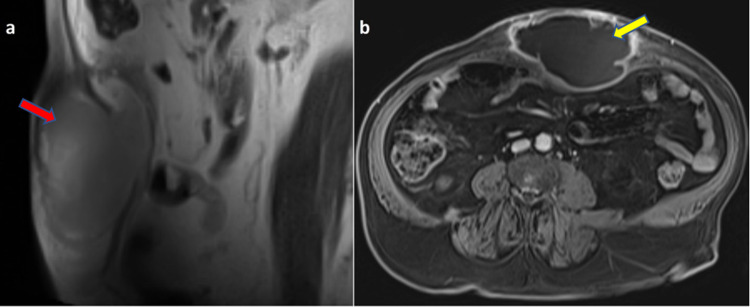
a) T2-weighted MRI sagittal plane showing a well-defined cystic lesion in the anterior abdominal wall (red arrow). b) T1-weighted post-contrast axial image showing midline cystic lesion in the anterior abdominal wall with irregular enhancing peripheral wall and few septations within (yellow arrow).
